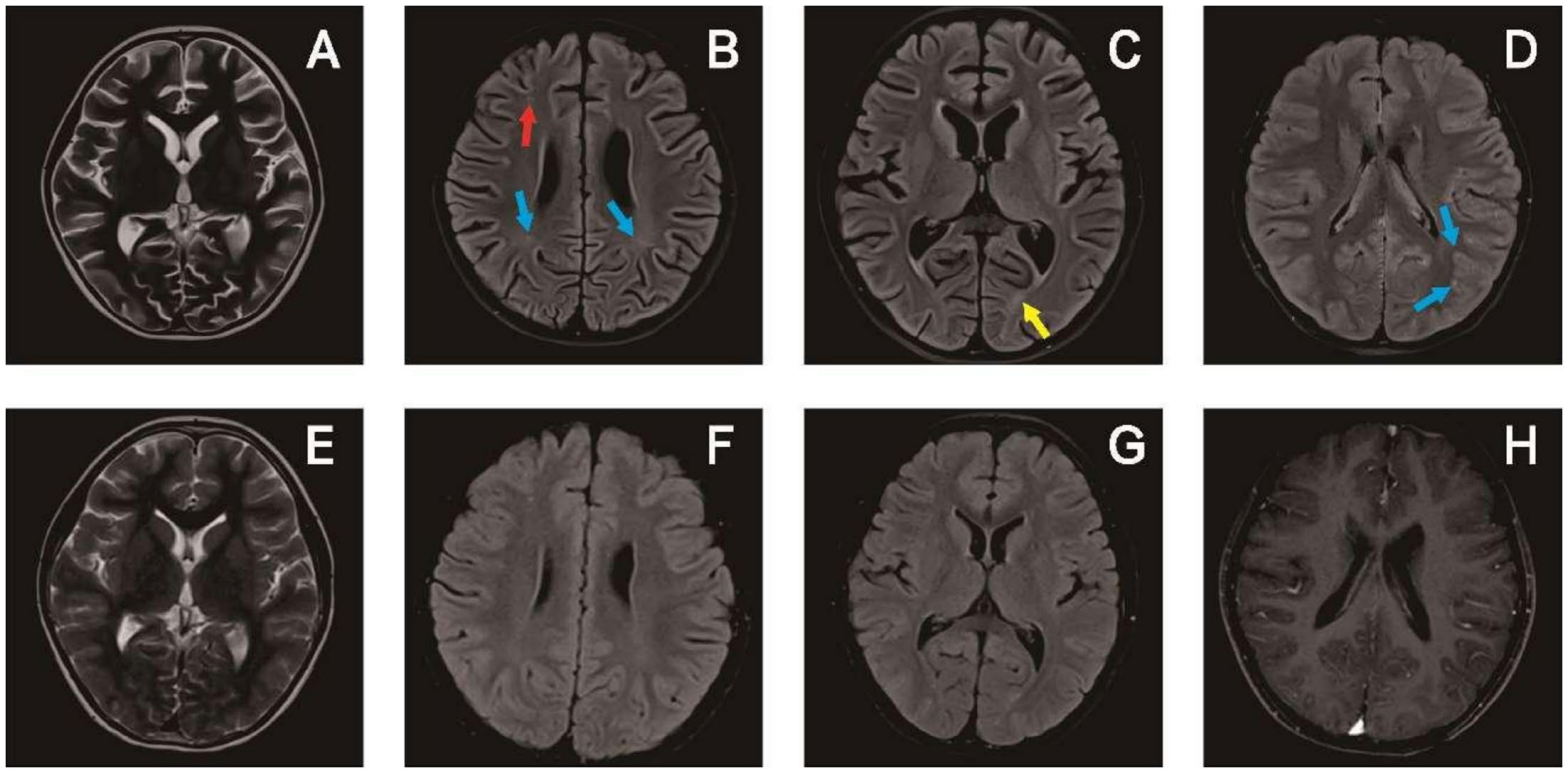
Brain MRI of patient 14 in Table 1: upon admission (A–D) and 1 year later (E–H). (A) T2 image showed widening of the cerebral sulcus. (B) T2-hyperintense lesions in the white matter of the frontal lobe (red arrow) and parietal lobe (blue arrow). (C) T2-hyperintense lesions in the white matter of the occipital lobe (yellow arrow). (D) Enhancement of T2 flair showed small strip like high signal shadows in the sulci of the brain. (E–H) Follow-up images of improved T2 lesions and enhancement.
